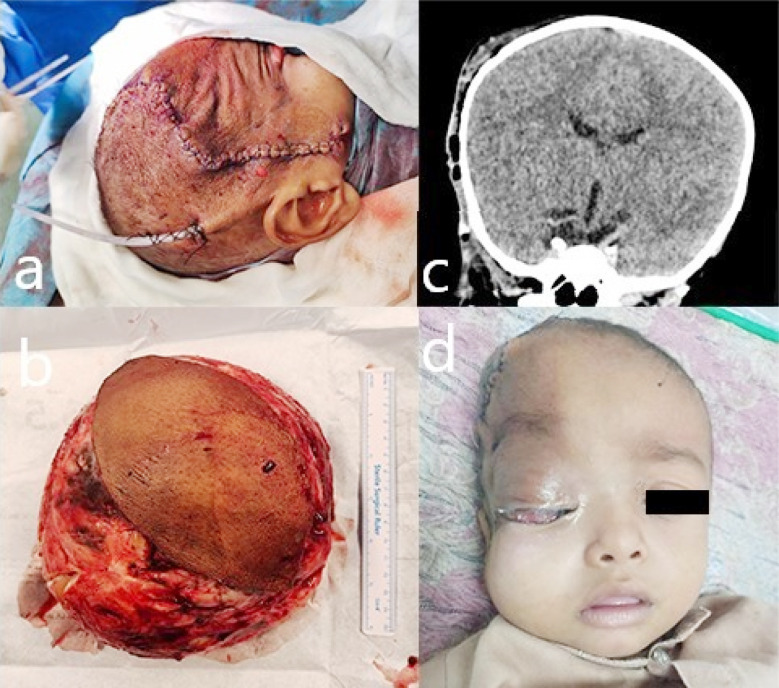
(a) Picture taken at OT table after skin closure and drain insertion. (b) A large tumor after resection. (c) Post-operative CT head coronal view showing edematous changes along the right temporoparietal region. (d) After 3^rd^ post-operative day, periorbital edematous changes are visible.
